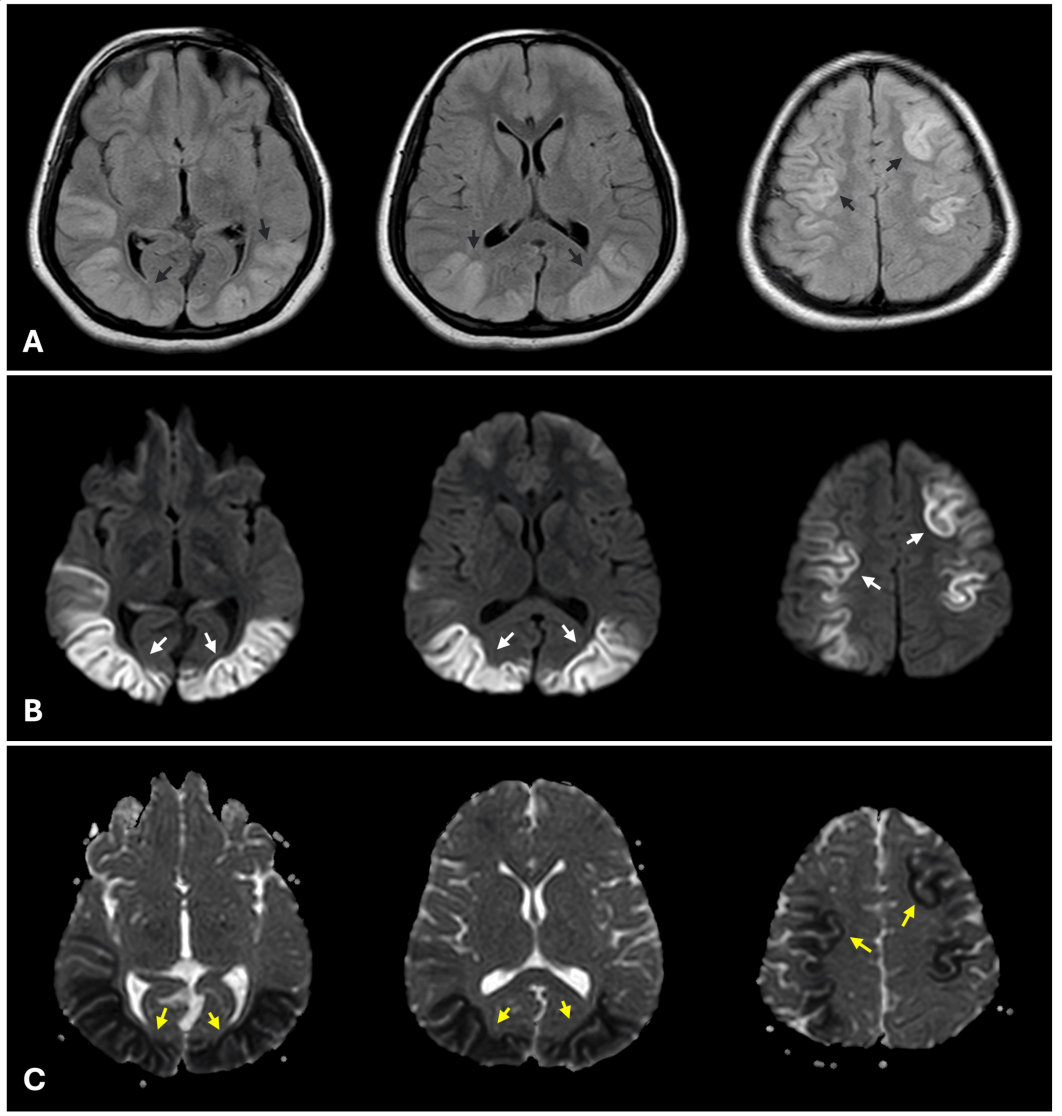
MRI findings on day 4. Axial FLAIR images (A) show extensive near-symmetrical bilateral occipital, with asymmetrical bilateral high parietal cortical gray matter hyperintensities (black arrows). Corresponding DWI (B) and ADC (C) sequences show restricted diffusion of the same regions (white and yellow arrows). ADC: apparent diffusion coefficient, DWI: diffusion-weighted imaging, FLAIR: fluid-attenuated inversion recovery, MRI: magnetic resonance imaging.
